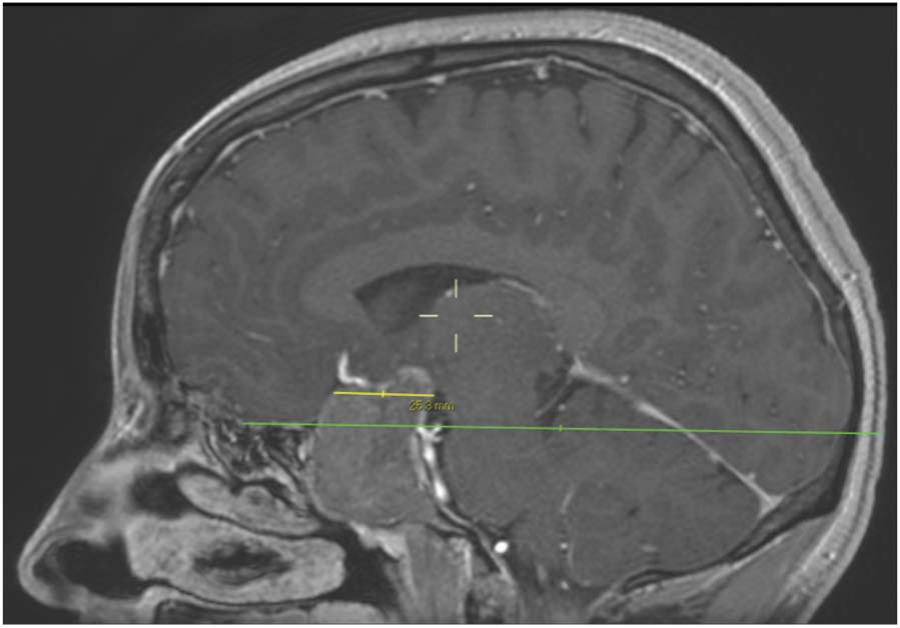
Contrast-enhanced sagittal T1-weighted MRI demonstrating a pituitary macroadenoma with suprasellar extension. The suprasellar anterior-posterior diameter (yellow line) is determined by measuring the longest anterior-posterior distance above and parallel to the planum line (green line).
